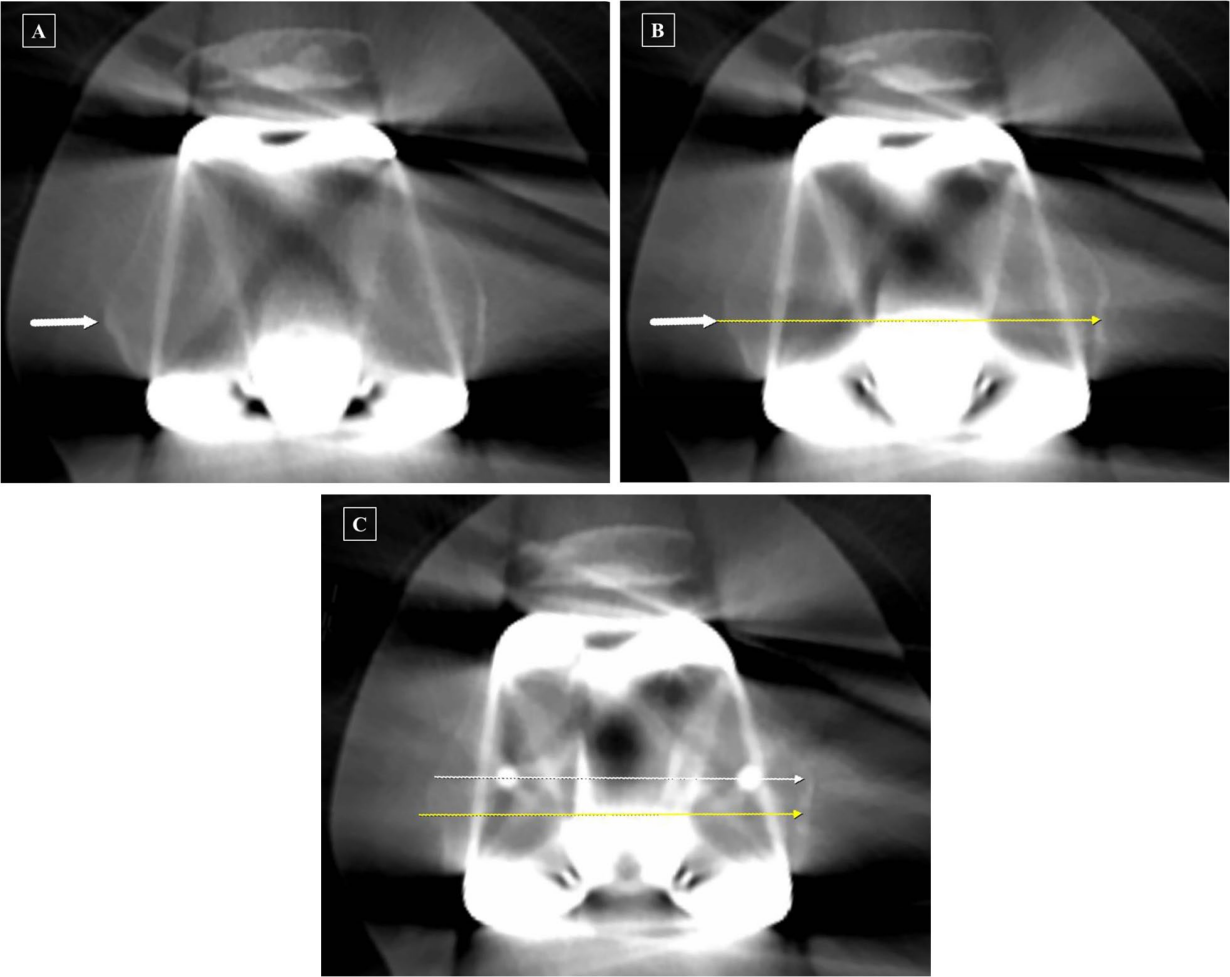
Radiographic determination of surgical transepicondylar axis (sTEA) and femoral-component rotational axis on the axial view of computer tomography. A White arrow: the tip of the lateral epicondyle. B Pasted white arrow: described above; the yellow line indicates the sTEA. C Pasted yellow line: described above; white line: the femoral-component rotational axis
